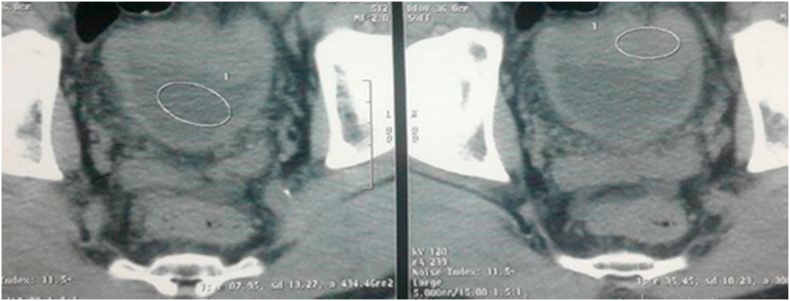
Computed tomography showed circumferential infiltration of the anterior bladder wall related to a tumor of the urachus.
